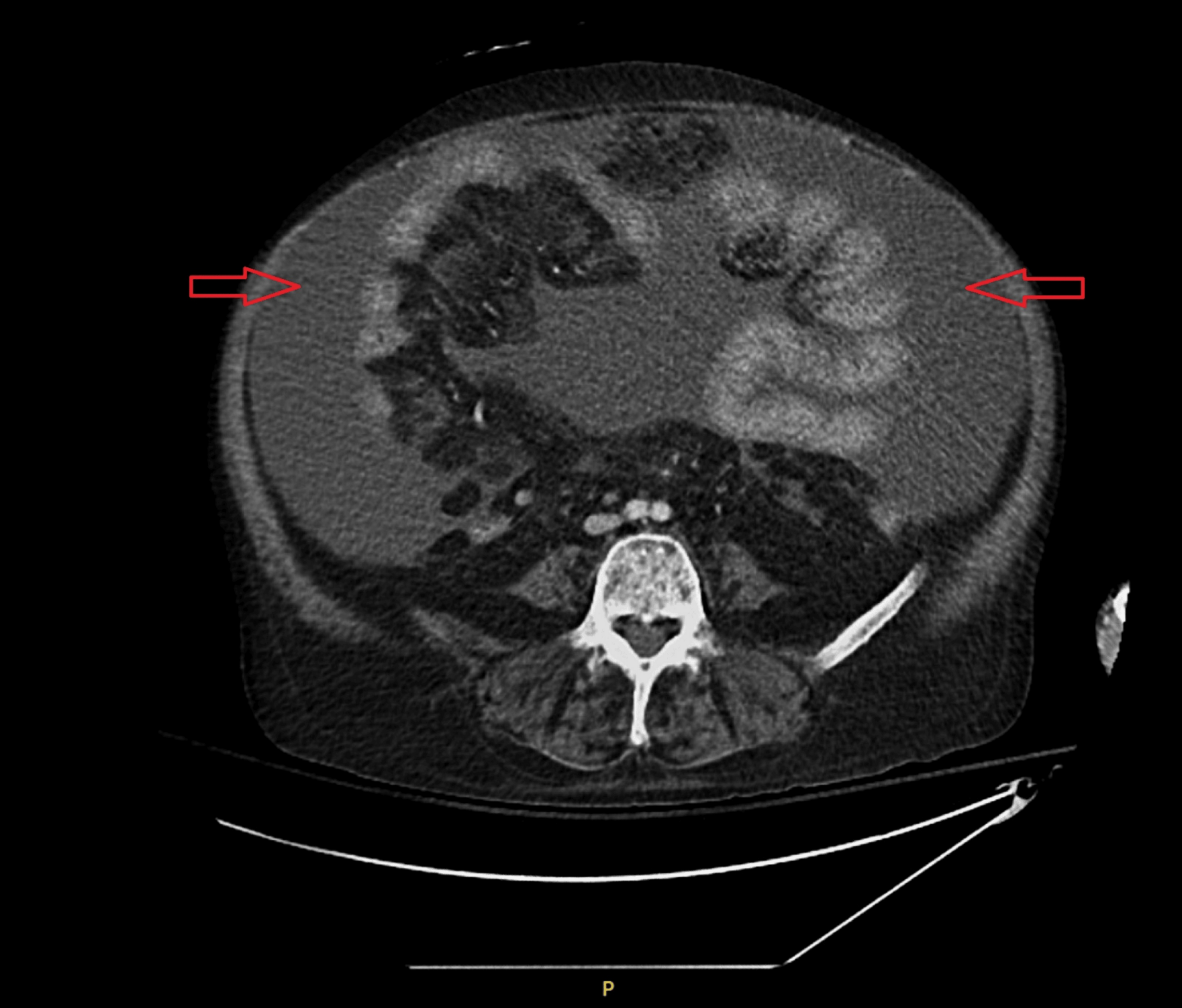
CT scan of the abdomen with moderate volume ascites (red arrows), morphologic changes of cirrhosis, and without evidence of pneumoperitoneum
